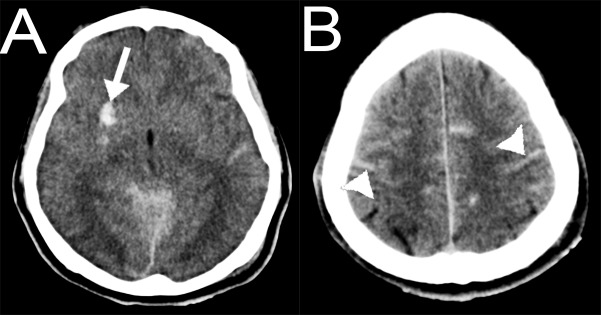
Case 2. Axial computed tomography (CT) of the head. (A) Right thalamic contusions (arrow) with (B) traumatic subarachnoid hemorrhage (arrowheads) are demonstrated.
